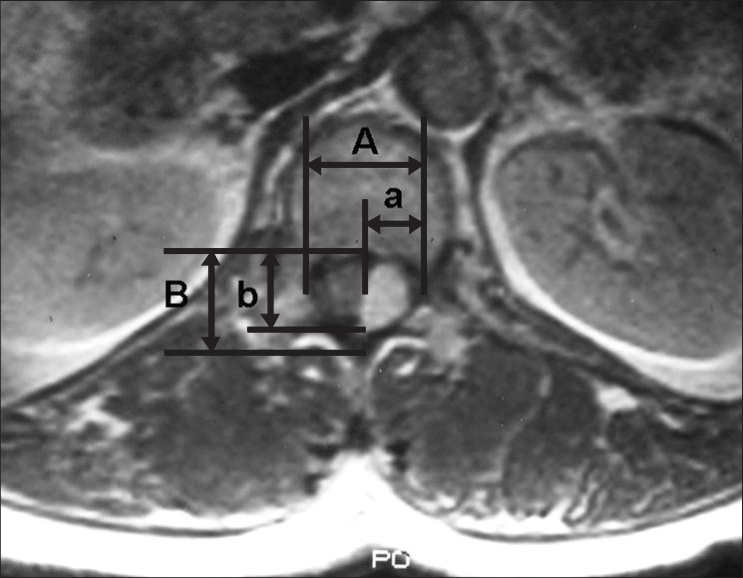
This picture shows how to calculate the percentage of tumor occupying the intradural space on an axial MRI film. It is as follows: {(a + b) / (A + B)} × 100. A: transverse diameter of the intradural space, B: longitudinal diameter of the intradural space, a: transverse diameter of the tumor mass, b: longitudinal diameter of the tumor mass.
